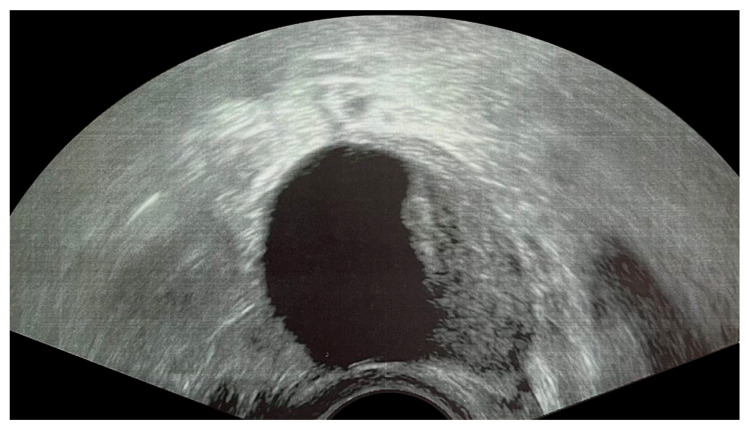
Ultrasonogram of an adolescent patient demonstrating a mature teratoma with a Rokitansky nodule that arises from the cyst wall and projects into the lumen.
